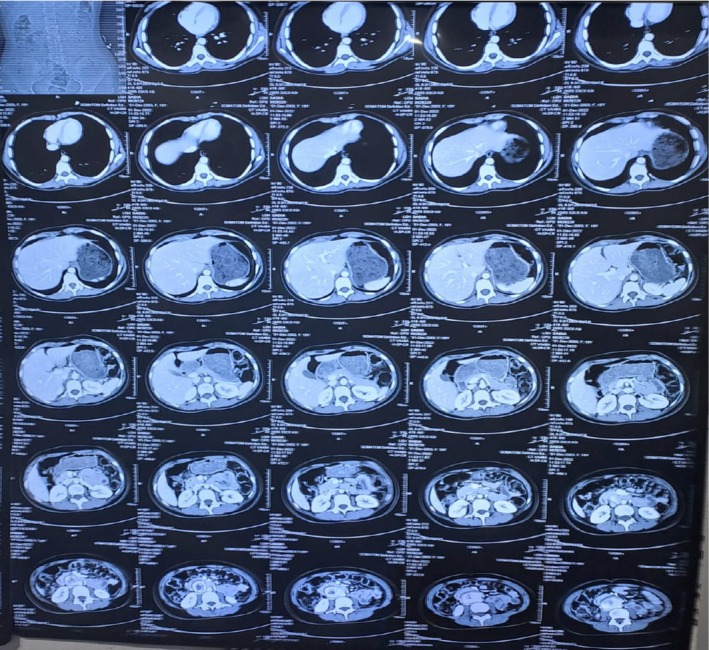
CT Abdomen with IV contrast showing the paraganglioma.
